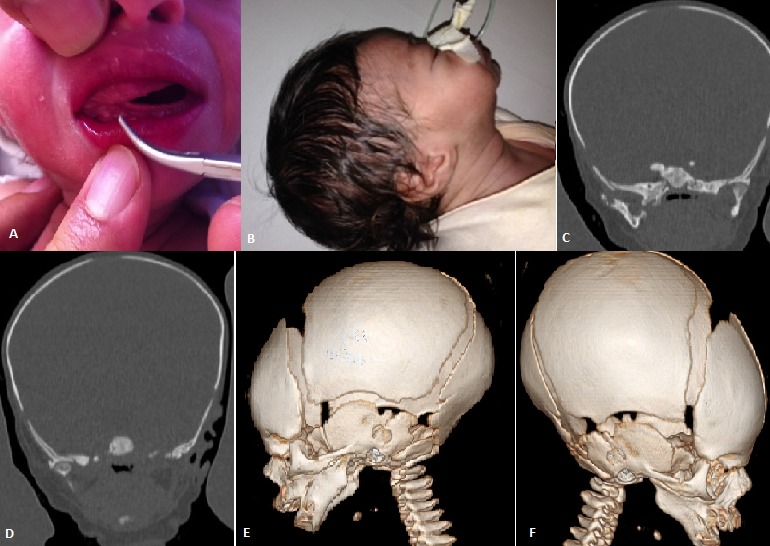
A)opening of the oral cavity limitation; B) retrognathia; C) Coronal CT scan image shows the left temporo-mandibular joint; D) Coronal CT scan image shows the right temporo-mandibular joint; E) Maxillary CT with 3D reconstruction revealed left Maxillomandibular synostosis; F) Maxillary CT with 3D reconstruction revealed right Maxillomandibular synostosis
